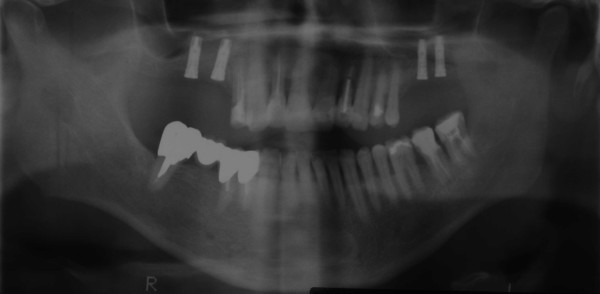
Panoramic radiograph five months after implant insertion.
